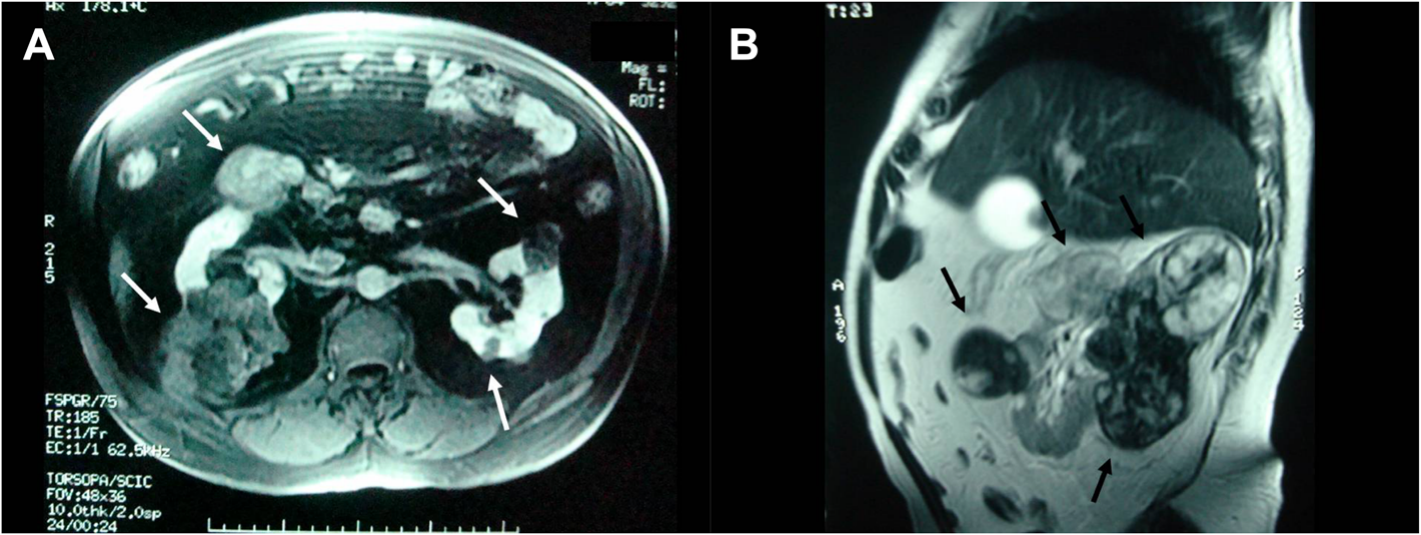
Magnetic resonance imaging (MRI) of the abdomen. a T1-weighted image with fat suppression after venous administration of the contrast medium. Several expansive formations can be observed in both kidneys with less intense enhancement than that of the adjacent renal parenchyma (white arrows). b T2-weighted image of the sagittal section of the right kidney. The black arrows show multiple expansive heterogeneous signal formations, with hyposignal and hypersignal areas in T2-weighted sequences and a 10-cm lesion in the lower pole of the right kidney
